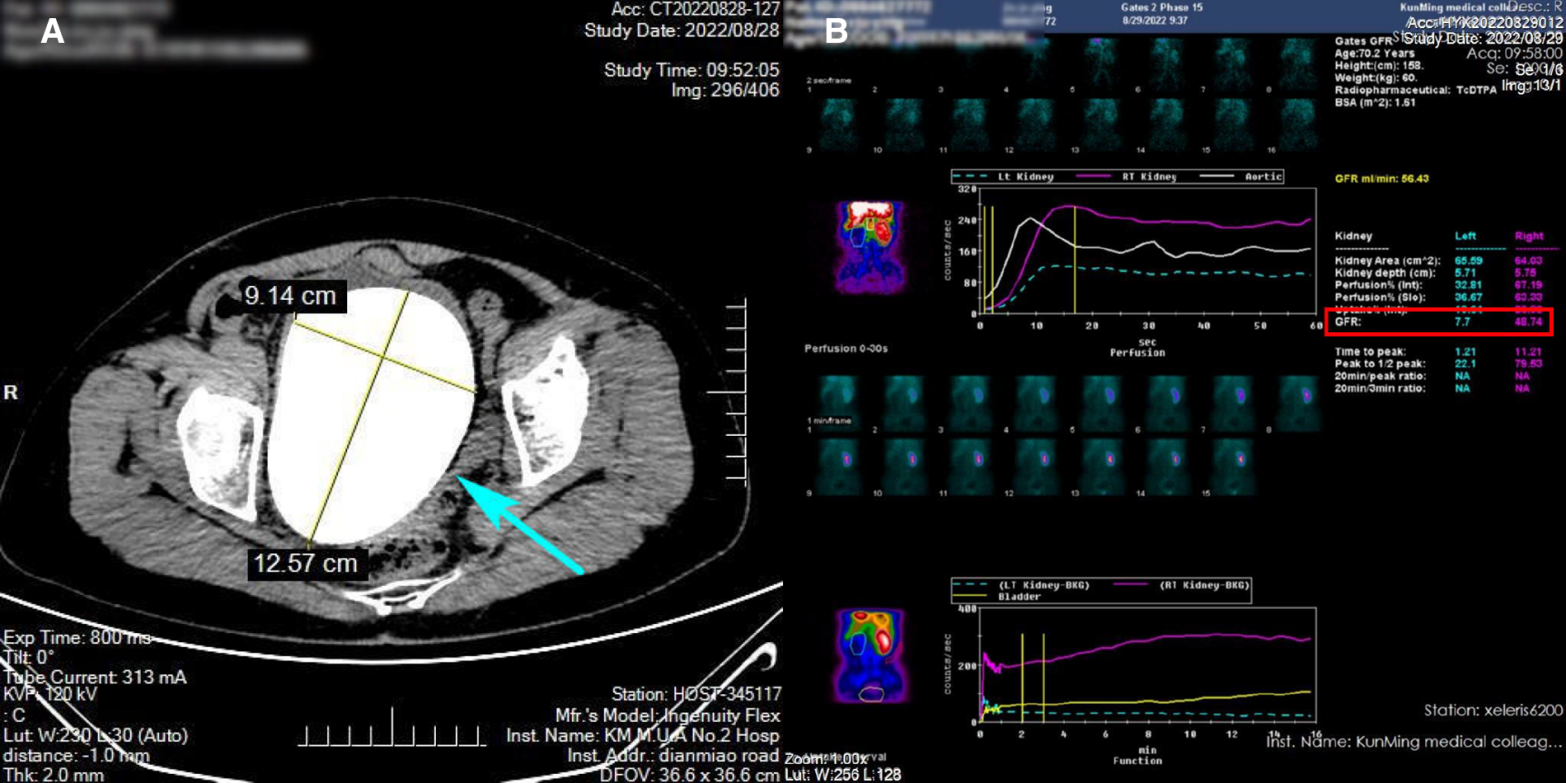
Computed tomography scan showing a neobladder stone (A, blue arrow). A renal scan showing poor blood perfusion in the left kidney (B).
